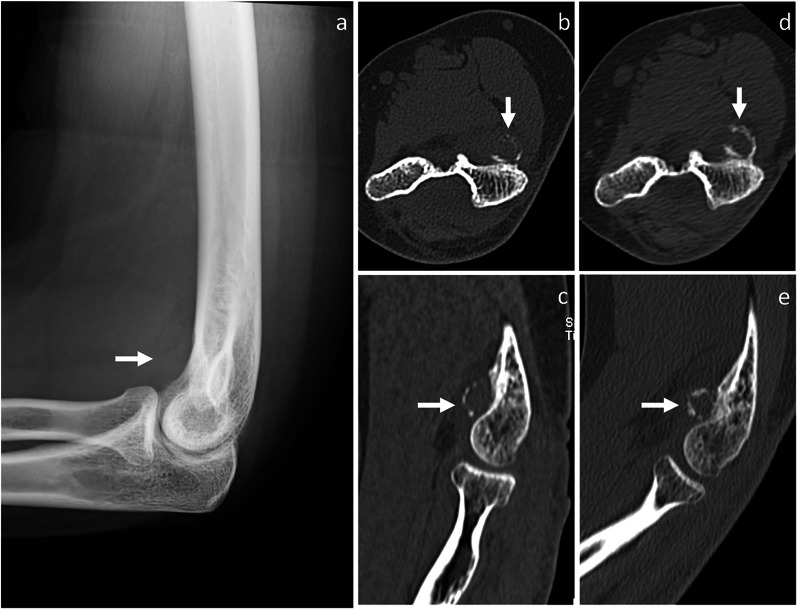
Florid reactive periostitis. On lateral X-rays view (a), a soft-tissue swelling is noted in contiguity with the volar aspect of the distal humerus. On axial (b) and sagittal (c) CT images, a partially mineralized mass with peripheral calcifications and mild periosteal thickening is noted. No cortical discontinuity is seen. Three months later, peripheral calcifications become more prominent, as shown in axial (d) and sagittal (e) CT images. Florid reactive periostitis ossificans was pathologically proven. Arrow points at the lesion in all images
